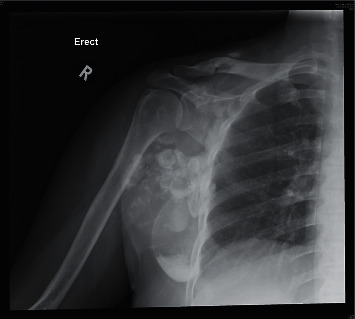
Radiograph of the right shoulder 1 year after teriparatide therapy.
